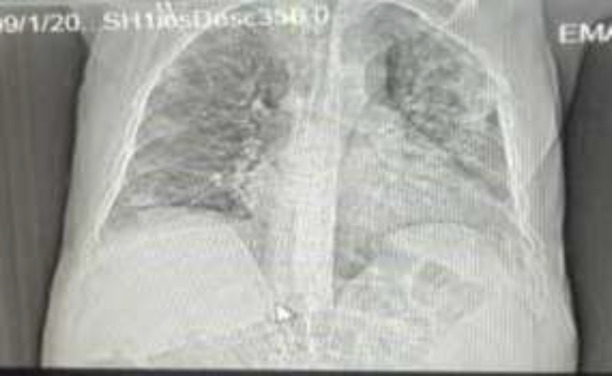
Chest x-ray of the patient with COVID-19 infection
